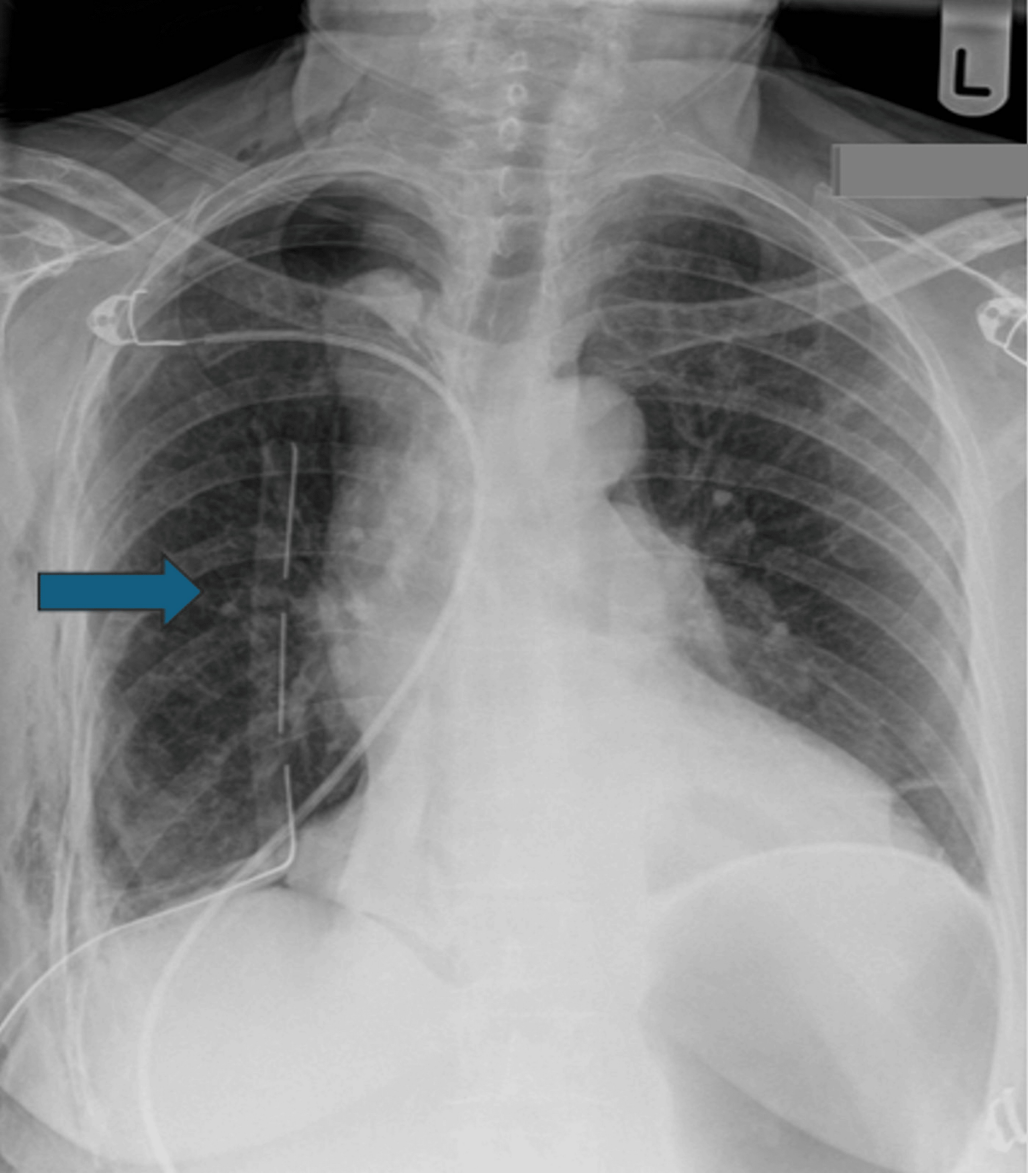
CXR showing postoperative expanded lung and drain in correct place CXR: chest radiograph
